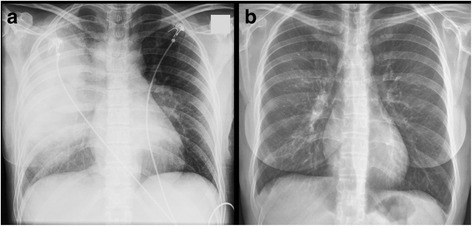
a Chest X-ray at time of presentation at the emergency department showing a large right-sided lobar infiltrate. b Chest X-ray at the outpatient department taken 42 days after initial presentation showing almost complete resolution of the infiltrate
